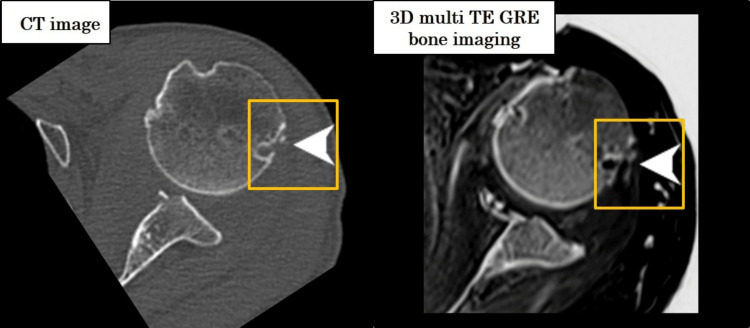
Clinical case: Hill-Sachs lesion CT-like MRI clearly visualizes the humeral head impaction fracture.
